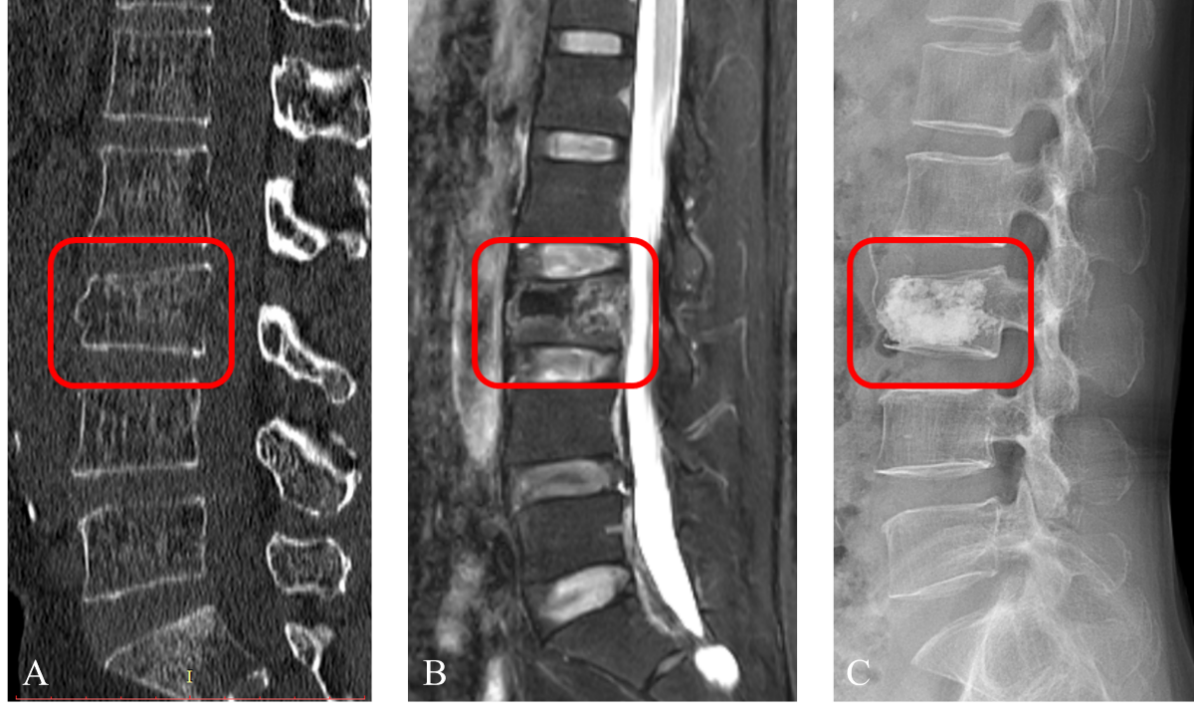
A case from the prospective validation cohort involves a 60-year-old female patient with osteoporotic vertebral fractures and an Assessment System of Thoracolumbar Osteoporotic Fractures score of 6. It was misclassified by the ImageNet model, but was correctly classified by the RadImageNet model. (A) Computed tomography imaging; (B) MRI imaging; (C) Postpercutaneous vertebroplasty showing bone cement leakage.
